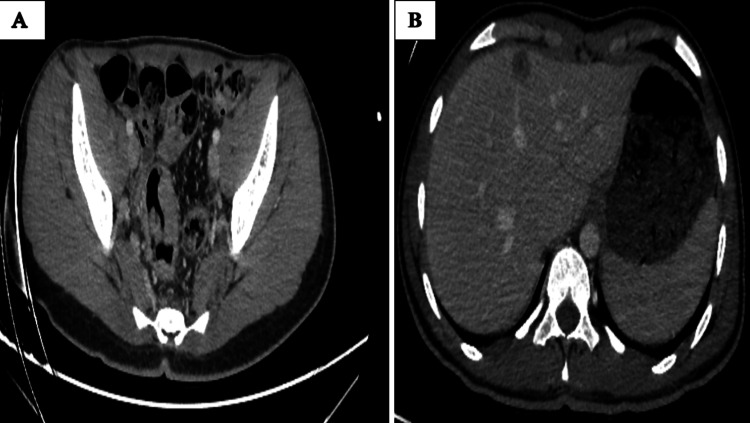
Axial contrast-enhanced CT images of the patient showing the circumferential thickening of the rectosigmoid junction (A) with two hypodense target lesions in hepatic segments VI (12 mm) and IV (15 mm), demonstrating mild enhancement after contrast injection (B)
